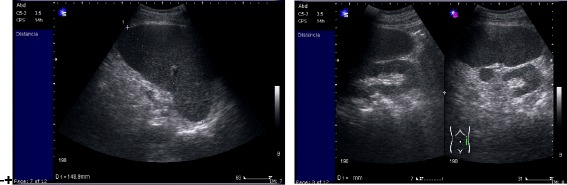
Upper abdominal ultrasound
